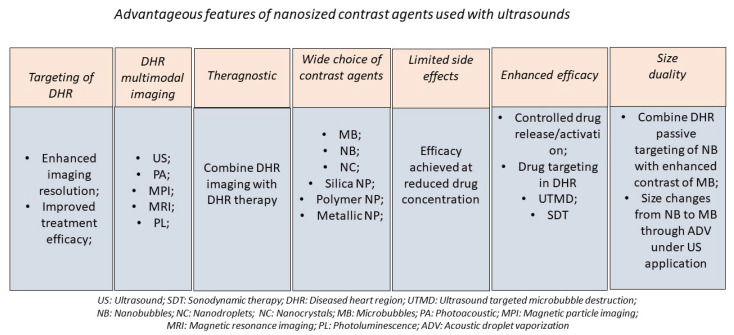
A list of advantageous features of nanosized contrast agents used with ultrasounds comprising: (i) the targeting of the diseased heart region resulting in the imaging/treatment of this region; (ii) the possibility to carry out multimodal imaging (US; PA; MPI; MRI; PL) and hence to benefit from the combined advantages of these different imaging methods; (iii) theragnostic properties (i.e., imaging and therapy can be combined); (iv) a wide choice of contrast agents is available including MBs, NBs, NDs, NCs, Silica NMs; Au NMs; (v) the efficacy of the treatment against heart diseases can be achieved at reduced drug concentration, hence minimizing the potential side effects of such drugs; (vi) the heart disease drug can be released/activated under the controlled condition by deciding to apply the ultrasound or not and by applying the ultrasound in the diseased heart region; (vi) the size of some NMs can be tuned (i.e., NDs can be transformed into MBs under acoustic droplet vaporization), hence enabling such system to benefit from the enhanced targeting efficacy of NDs (i.e., the EPR (enhanced permeability and retention) effect was improved for NDs of relatively small sizes and MBs yielded superior echogenicity).
